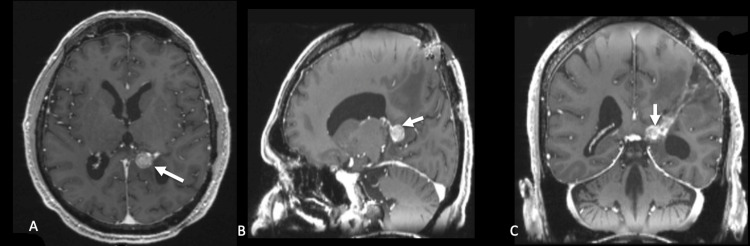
Follow-up MRI (A) Axial, (B) sagittal, and (C) coronal post-contrast T1-weighted images show a newly developed enhancing nodular lesion in the left lateral ventricle atrium (white arrows), consistent with local tumor recurrence five months after surgery.
